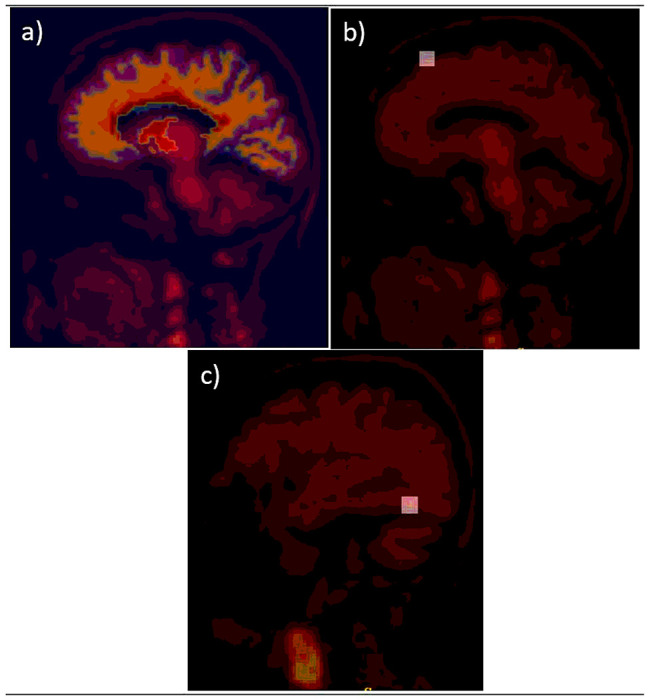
Example of inserted FreeSurfer brain atlas generated using T1 MRI brain image (a) and spherical lesions (b) and (c). The inserted lesions and atlas are superposed on the PET image.
